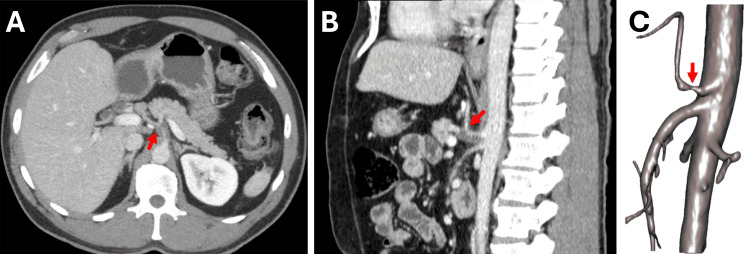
Contrast-Enhanced Computed Tomography Angiography A: Axial view, red arrow indicating the celiac trunk with a thrombosed false lumen and a patent true lumen. B: Sagittal view, red arrow indicating the celiac trunk with a thrombosed false lumen and a patent true lumen. C: Three-dimensional reconstruction, red arrow indicating narrowing of the true lumen.
